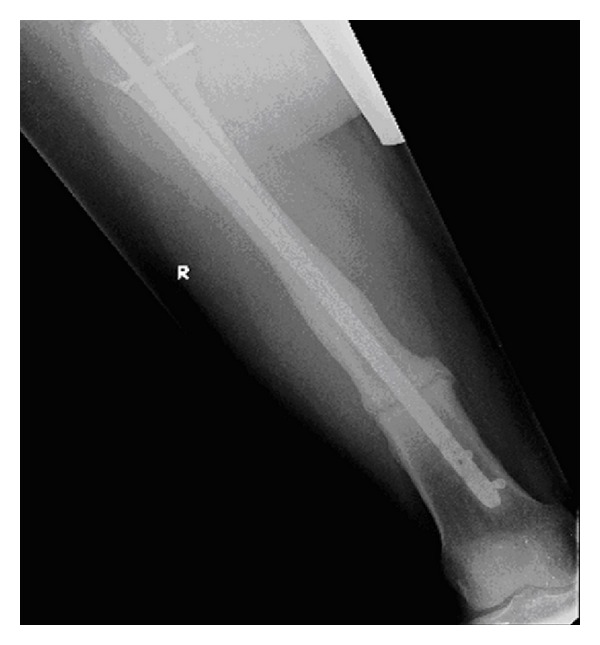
AP radiograph following corrective osteotomy of the right femur.
